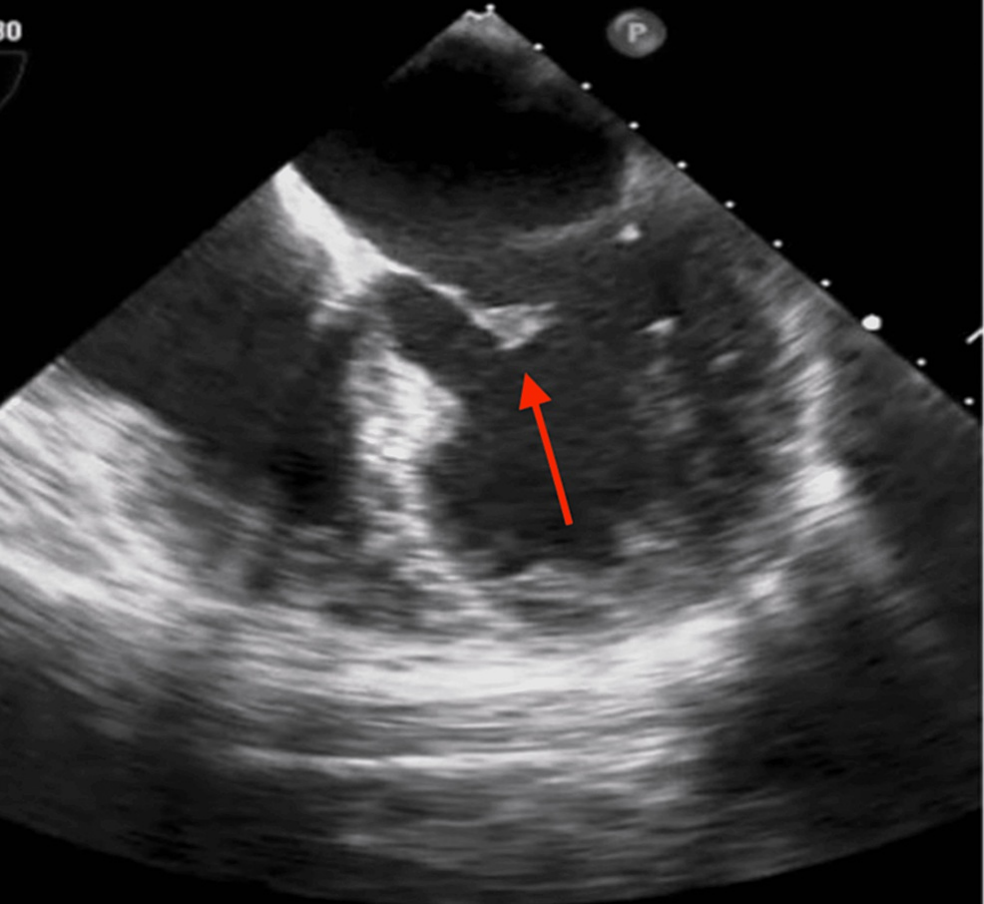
Transesophageal echocardiogram demonstrating a 5mm by 3mm anterior mitral valve vegetation
